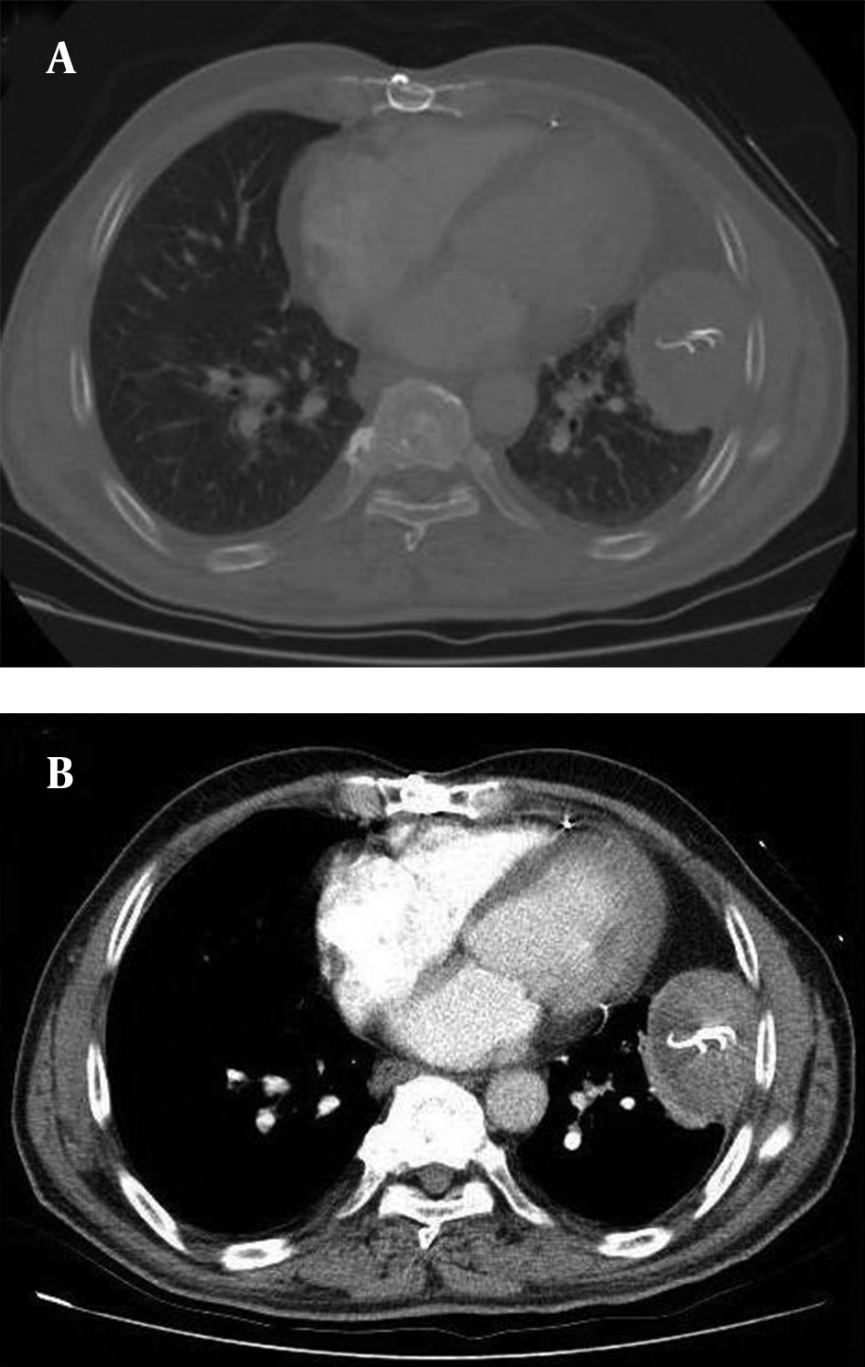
A) Parenchymal and B) Mediastinal windows of thorax CT scan revealed a pleural-based mass with a hyperdense rim capsule in the laterobasal segment of the left lower lobe with hyperdense striped appearance in the center
